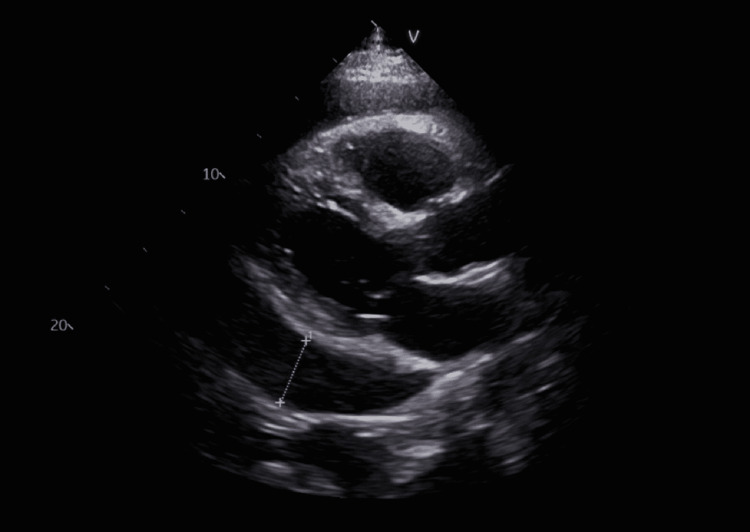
Echocardiogram: parasternal long-axis view White line: pericardial effusion
